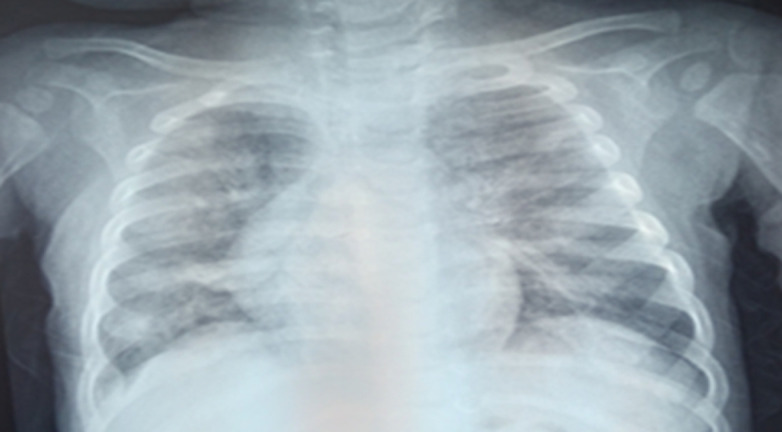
radiographie du thorax montrant des opacités alvéolo-interstitielles bilatérales
